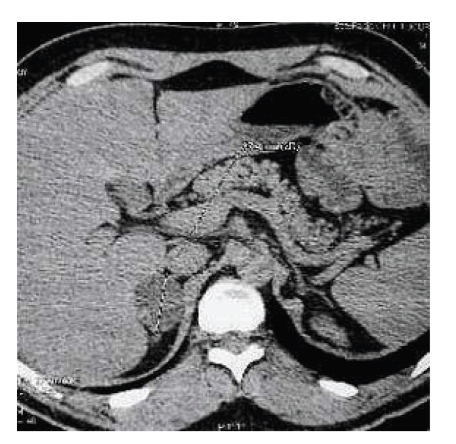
CT scan of the abdomen demonstrating a 2.5 × 4.0 cm mass in the right adrenal gland.
